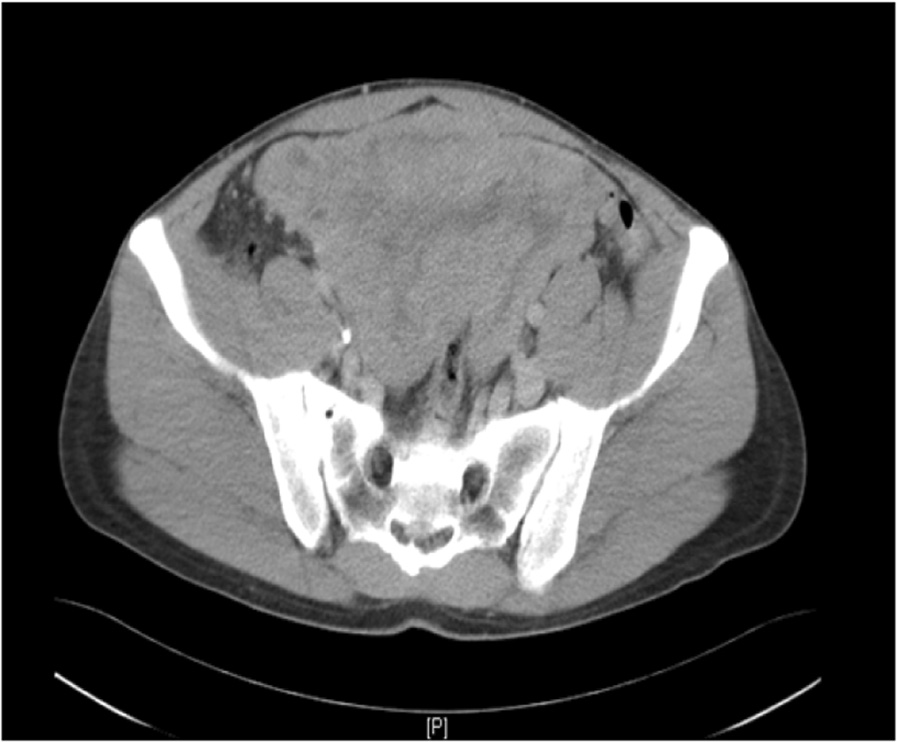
CT scan of the patient. GIST of the small bowel 18 x 10 cm in size when it was first diagnosed prior nedoadjuvant treatment.
